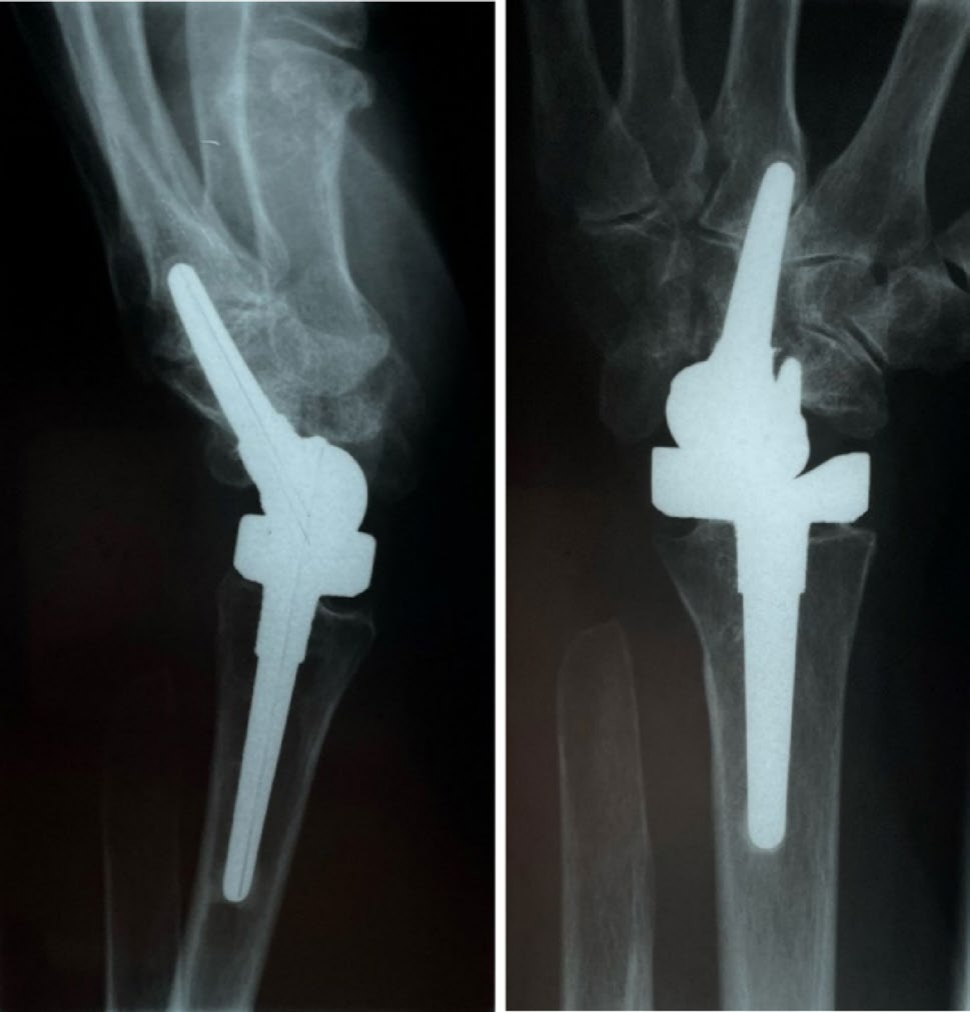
Case 1 - Left postoperative X-ray in lateral and a.p. view
